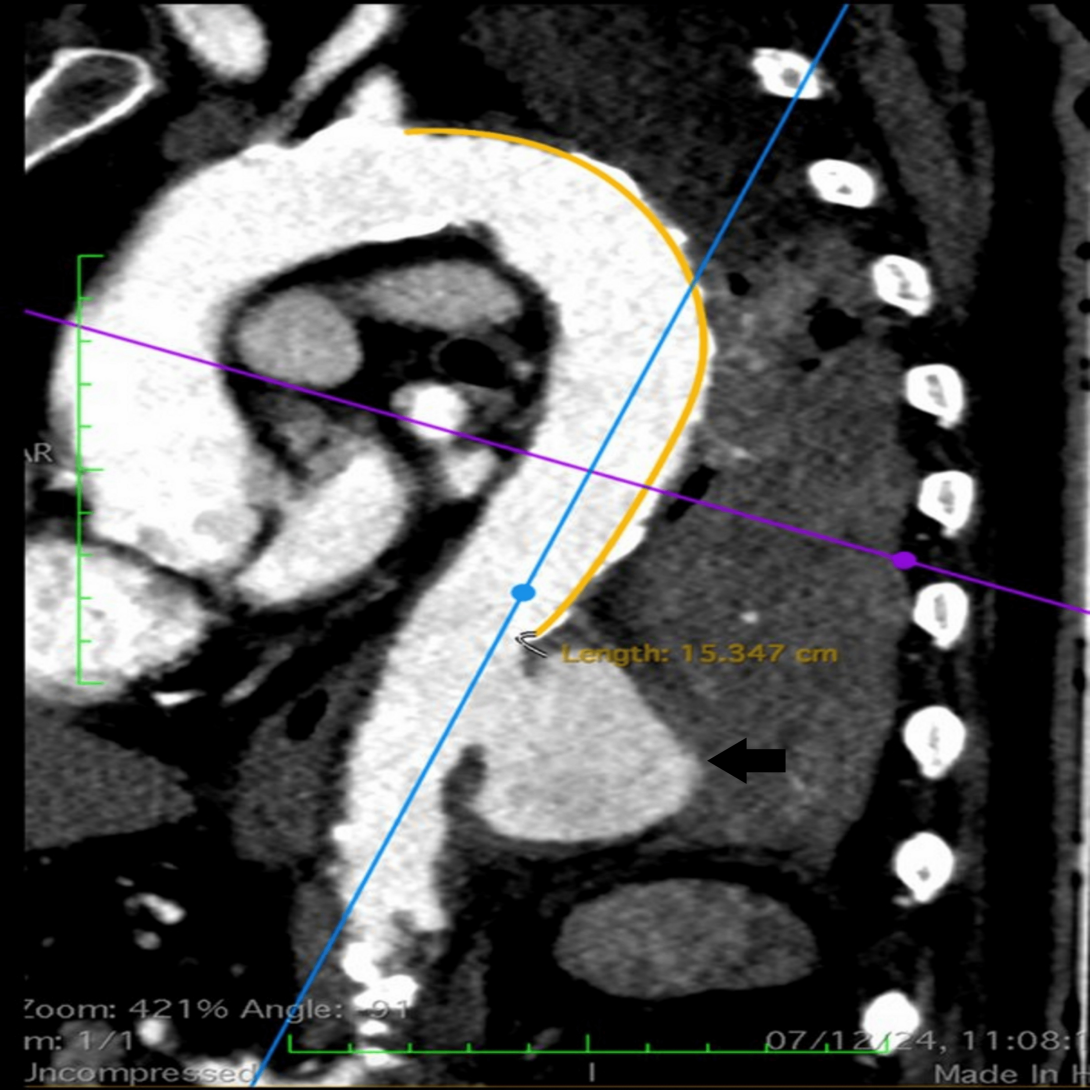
CT aortogram showing a 40 mm saccular aneurysm in the descending thoracic aorta, adjacent to the left lung parenchyma, which was compressing the left lung, causing uncontrollable bloody sputum (black arrow pointing to the saccular aneurysm).
